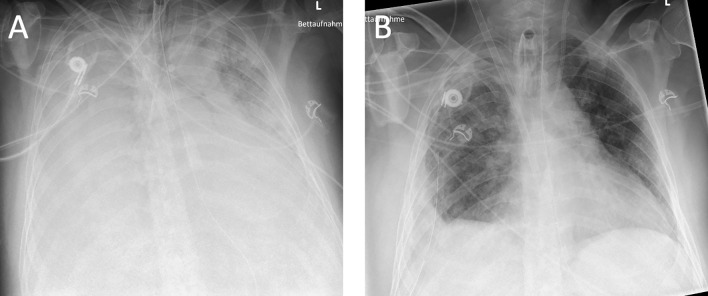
Chest X-ray of the patient immediately before interferon administration at week 4 (A) and after administration and clinical improvement at week 6 (B).
